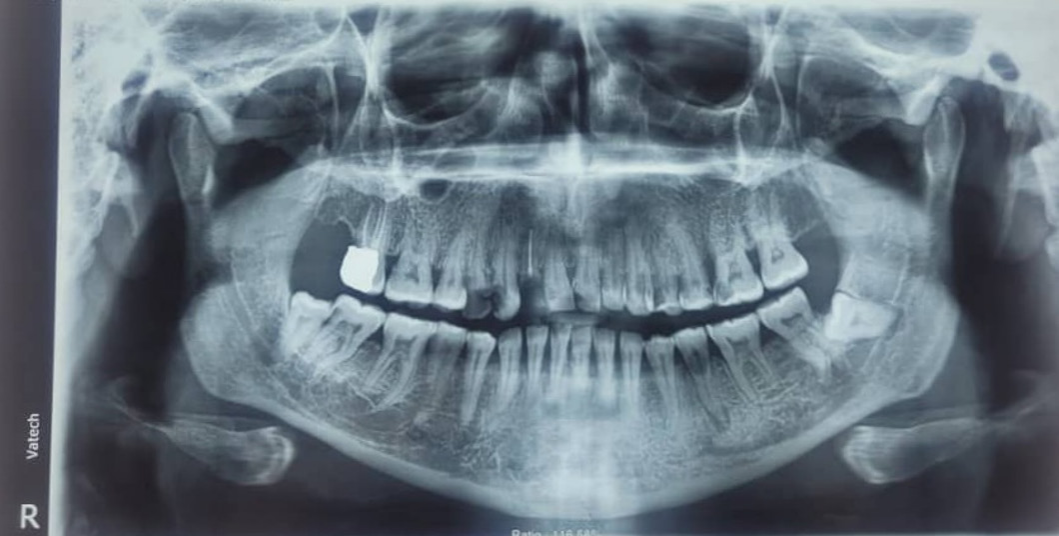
Baseline radiography before the root canal treatment.
